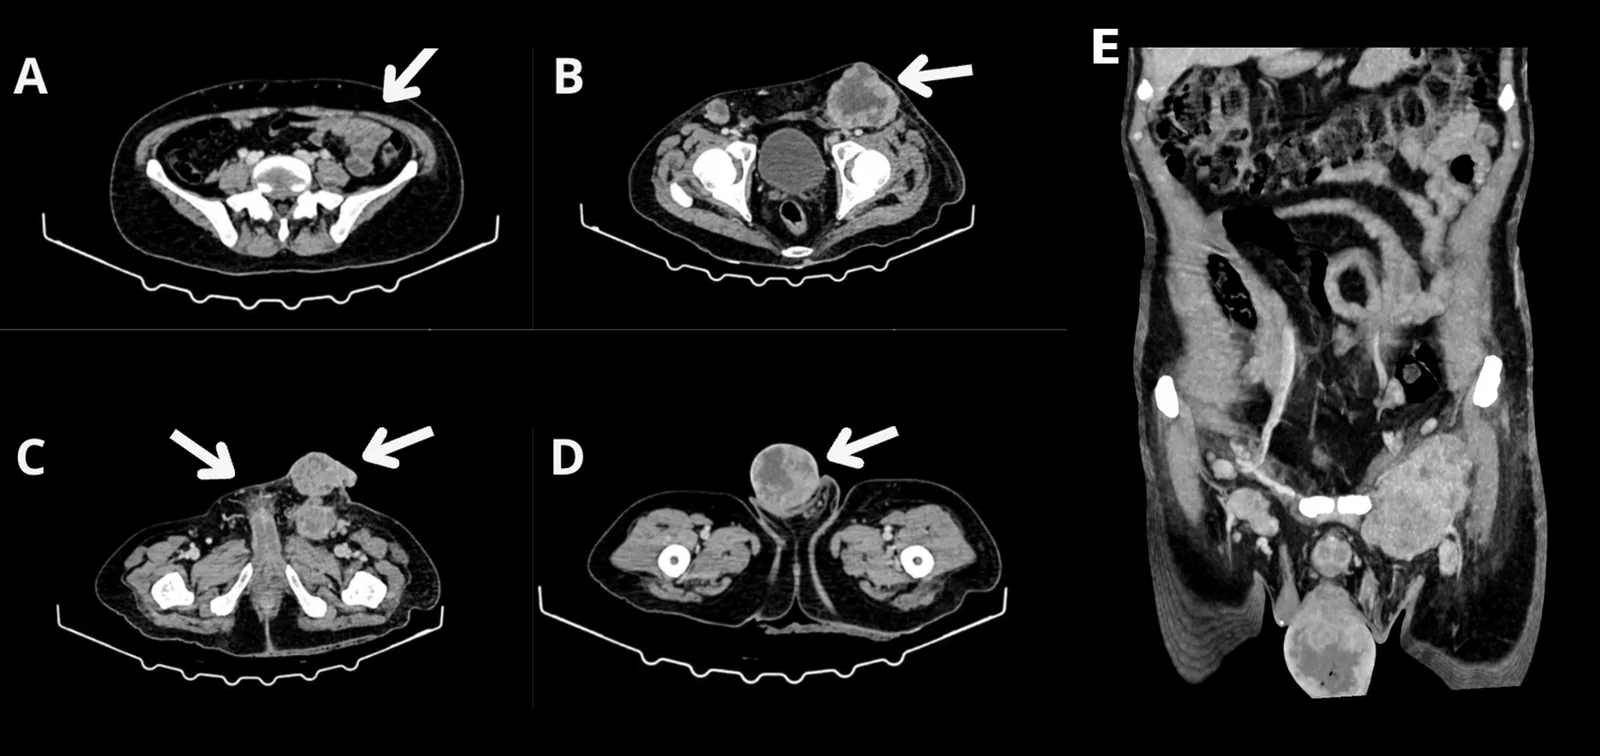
CT findings at restaging. (A) Lymphadenopathy at the left iliac bifurcation. (B) Bulky left-sided nodal disease. (C) Left inguinal nodal disease associated with penile involvement. (D) Penile tumoral infiltration. (E) Bulky left inguinal tumor mass with pelvic extension, also involving the penis and infiltrating the scrotal region. Arrows indicate the main sites of disease involvement. CT: Computed tomography.
